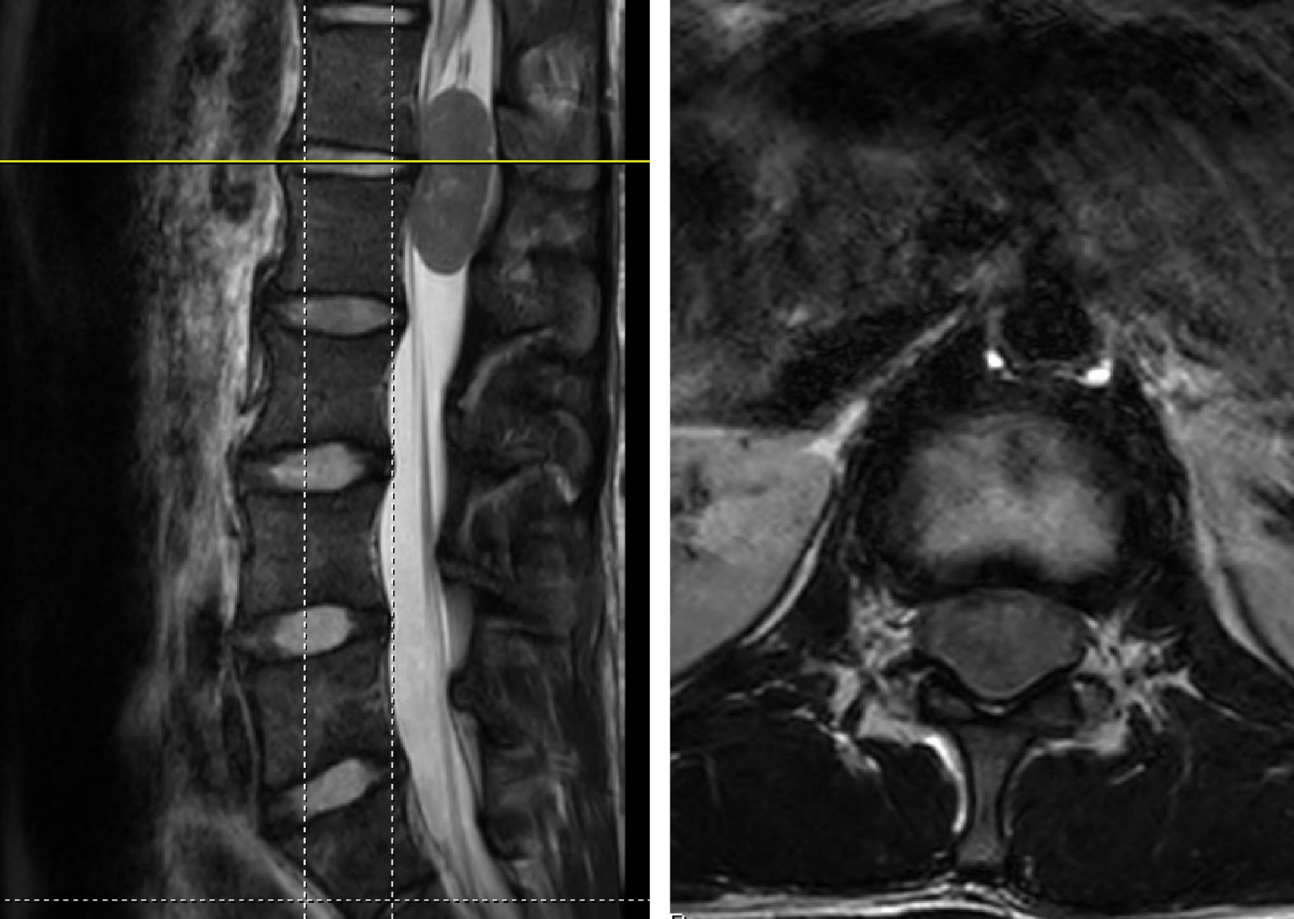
Preoperative MRI lumbar spine without contrast—T2 sagittal and axial sequences (left and right, respectively)—demonstrating an intradural extramedullary lesion centered between the L1-L2 vertebral bodies
